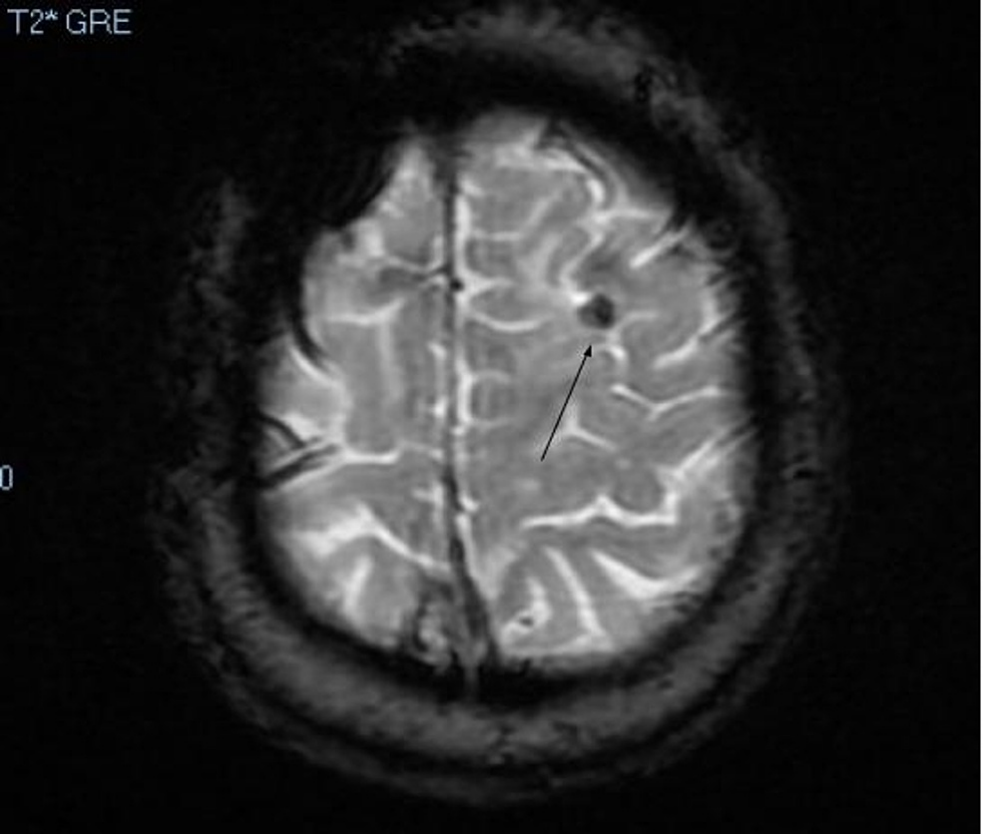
Axial brain MRI gradient echo sequence showing that the lesion (black arrow) appears as a dark lesion, indicating a calcium-containing object.
